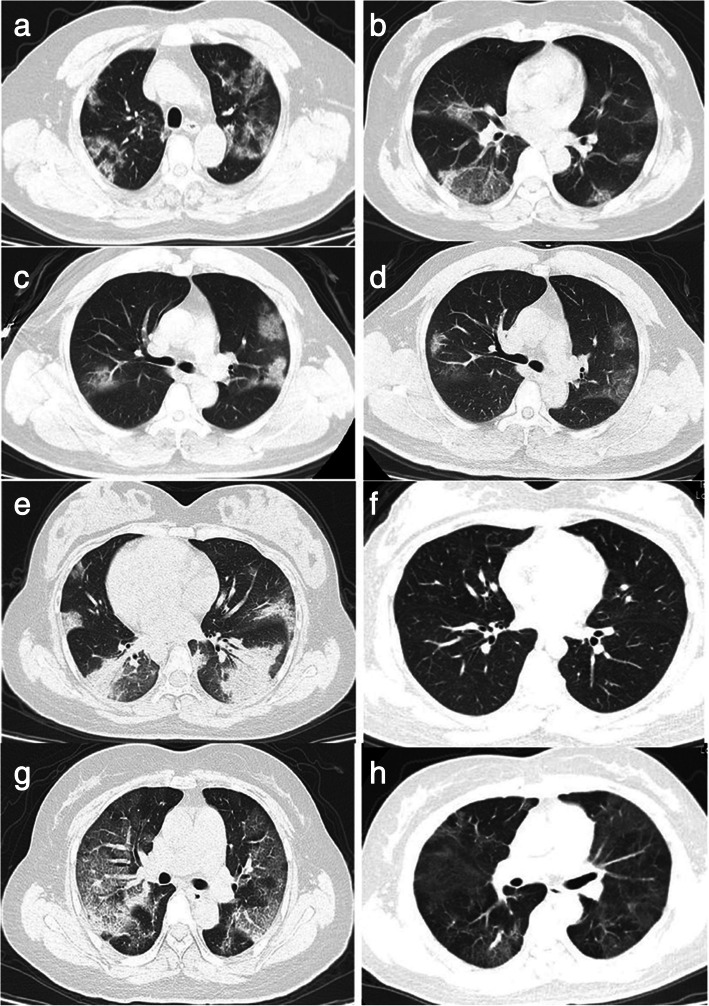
CT features of coronavirus disease-2019 patients. a. CT images of a 45-year-old female patient showing multiple ground-glass shadows in both lungs that were partially fused; b. CT images of a 42-year-old male patient showing a crazy-paving pattern under the pleura of the right lung; c–d. A 56-year-old female patient’s CT reviewed 7 days later showing that the original consolidation of both lungs was diminished, another ground-glass-like density shadow appeared in the middle lobe of the right lung, and the lesions in the lungs showed “wandering” characteristics; e–f. A 32-year-old female patient was re-examined after 10 days of treatment. CT showing that the multiple lung lesions disappeared; g–h. A 68-year-old female patient was re-examined after 15 days of treatment. CT showing that the diffuse lesions of both lungs were absorbed into extensive fibrosis
